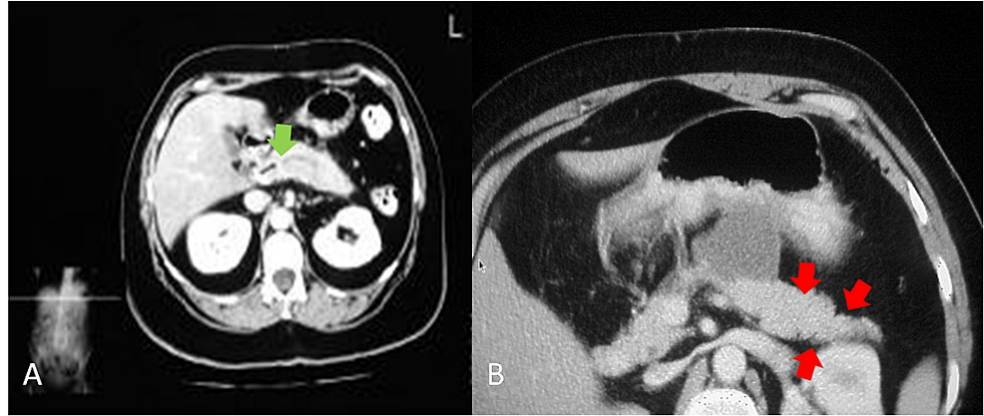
MRI of the abdomen with contrast A) diffuse slight dilatation of the main pancreatic duct with slightly prominent side branches most suggestive of chronic pancreatitis (green arrow); B) fat stranding surrounding the pancreatic body and tail (red arrows)
